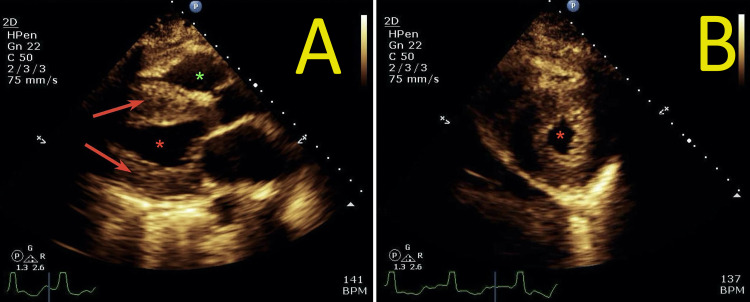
Transthoracic echocardiogram performed on the second day of admission Parasternal long-axis view (panel A) and parasternal short-axis view (panel B) Transthoracic echocardiogram demonstrated concentric left ventricular hypertrophy with the characteristic granular appearance of the myocardium. The red arrow indicates left ventricular myocardium. The red asterisk indicates the left ventricular cavity. The green asterisk indicates the right ventricular cavity
